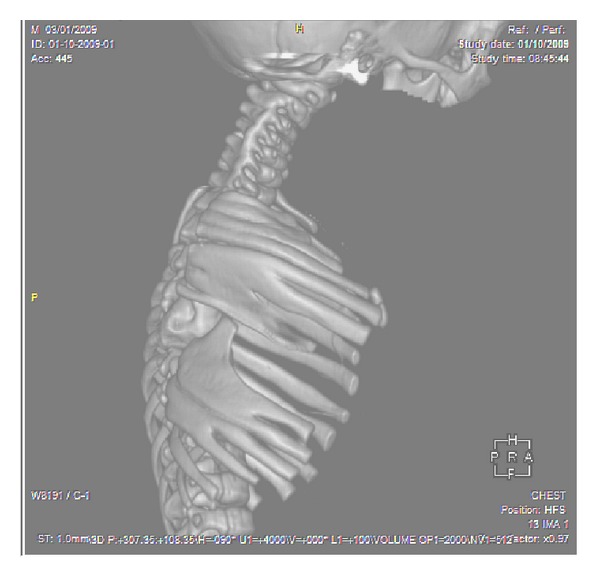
Three-dimensional CT scan lateral thoracic image demonstrating many rib points of fusion originated posteriorly.
